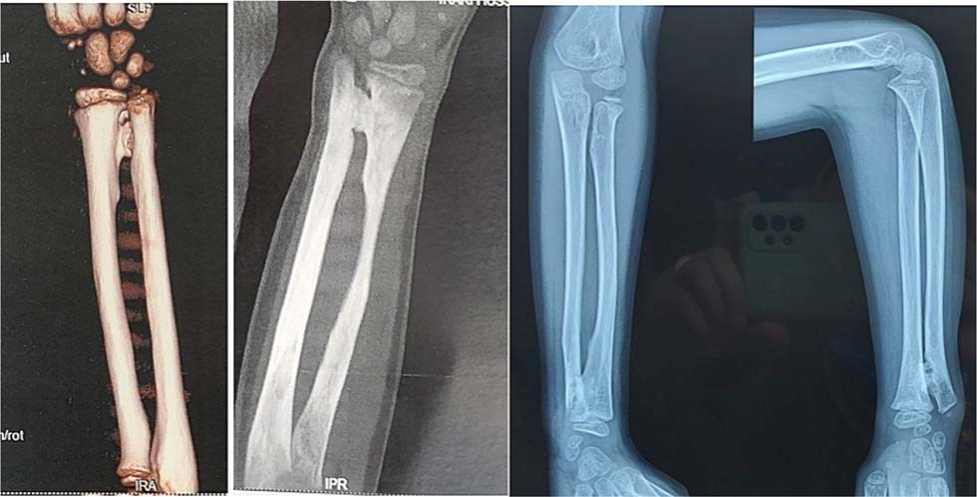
CT and X-ray images demonstrating distal radioulnar synostosis.
